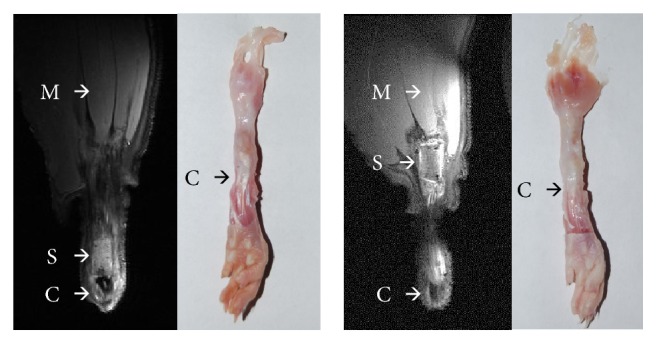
T_2_-weighted MR images and photographs (dorsal view) of experimental hind limbs repaired with collagen scaffolds after 28 days of implantation. Left MRI: scaffold is located distally as implanted and expected. Right MRI: scaffold is located proximally in the gastrocnemius muscle. Both prepared samples (for biomechanical testing) with analogues scaffold position gave no evidence about this position macroscopically. Arrows mark the position of calcaneus (C), scaffold (S), and gastrocnemius muscle (M).
